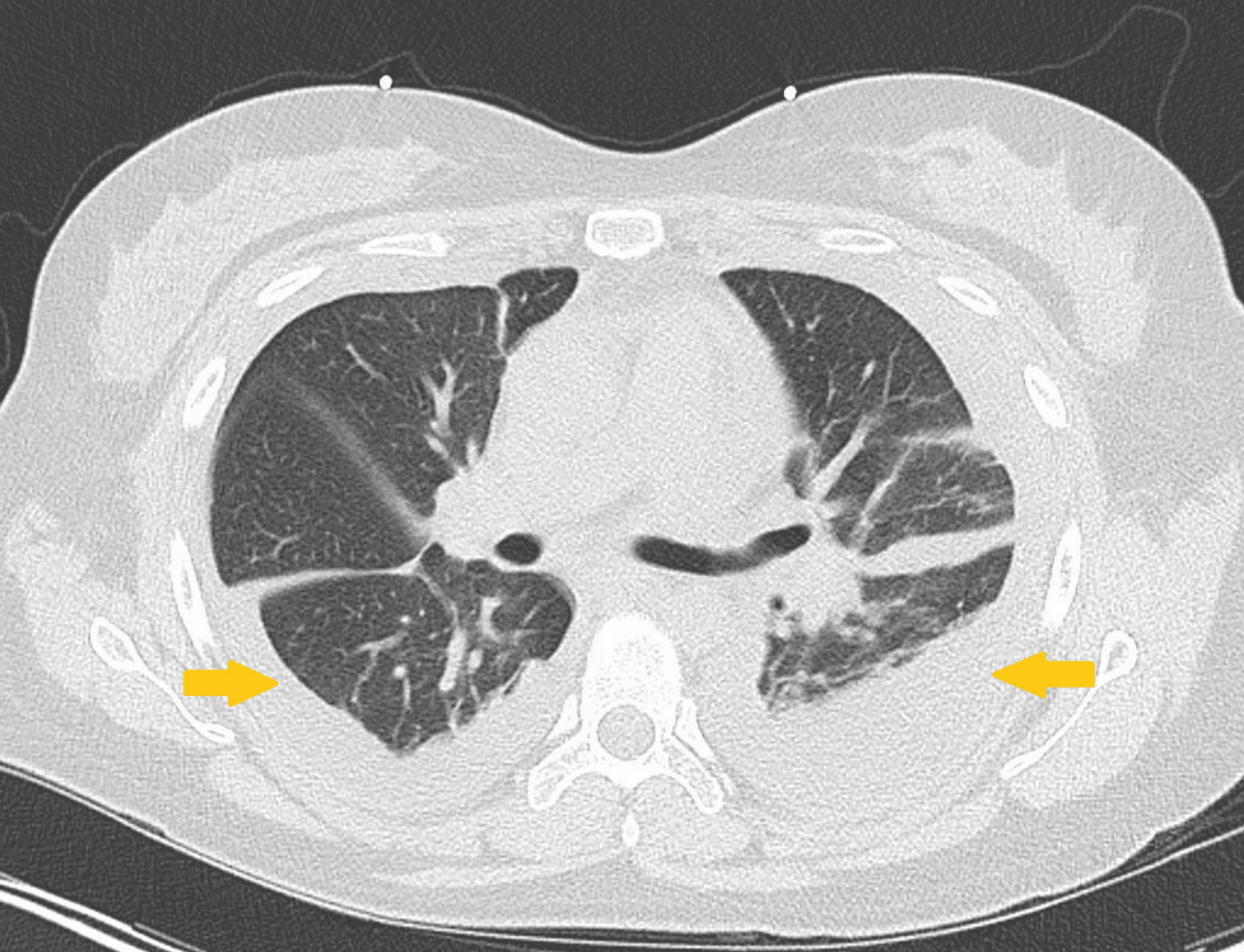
A CT of the chest without contrast was done on re-admission one month after initial hospitalization, showed worsening bilateral loculated pleural effusions.
